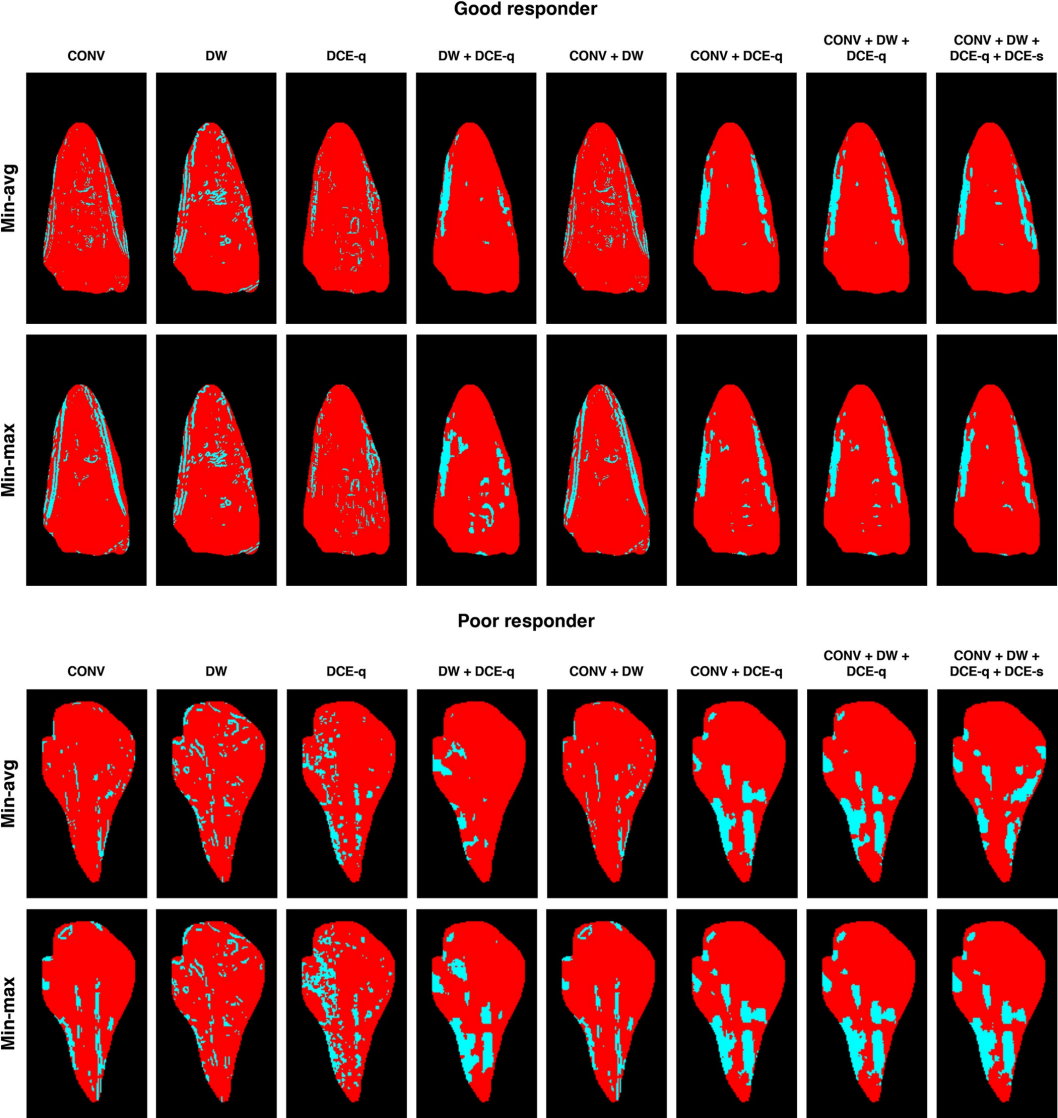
Viability mapping of tumor AOI computed using different combinations of weight optimization methods and MRI subsets. Tumor viability maps are shown for a good-responding patient (*N*_hist_^*ℓ*^ ≥ 90%) and a poor-responding patient (*N*_hist_^*ℓ*^ < 90%). Necrotic and viable tumor regions are represented in red and blue, respectively.
